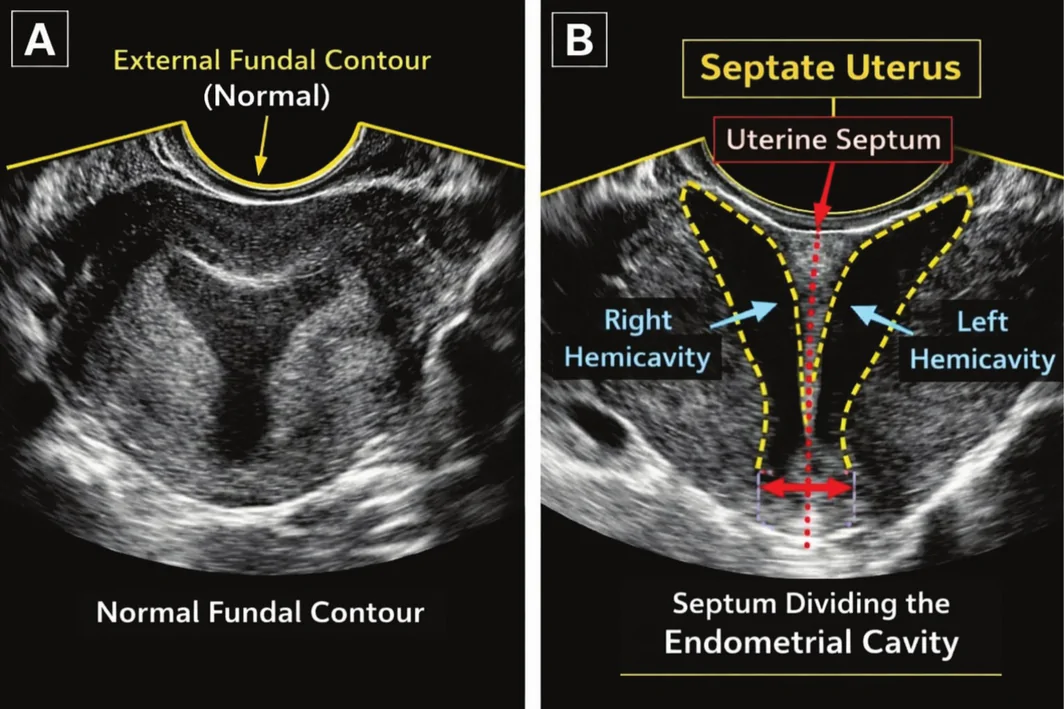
Transvaginal ultrasound demonstrating a septate uterus. (A) Transvaginal ultrasound image in the coronal plane showing the uterine cavity with a preserved, smooth external fundal contour and no fundal cleft, indicating a normally contoured uterine fundus. (B) Midline echogenic intrauterine septum (solid arrows) extending inferiorly from the fundus and dividing the endometrial cavity into two symmetric hemicavities (dashed lines). The intact external contour without fundal indentation supports a septate rather than bicornuate uterus (ASRM 2021 criteria).
